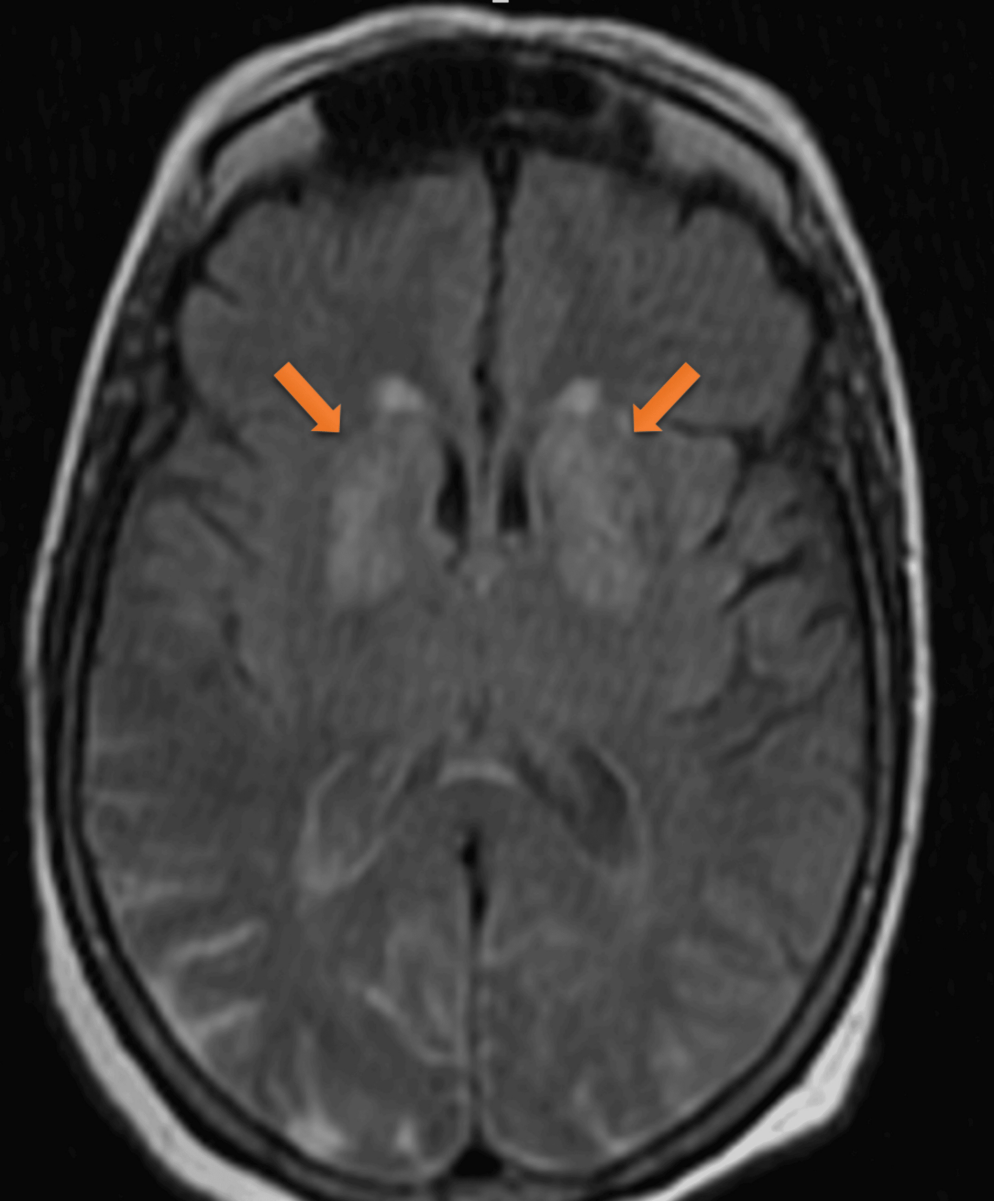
Axial MRI T2 revealing hyperintensities in the striatal region bilaterally MRI: magnetic resonance imaging
